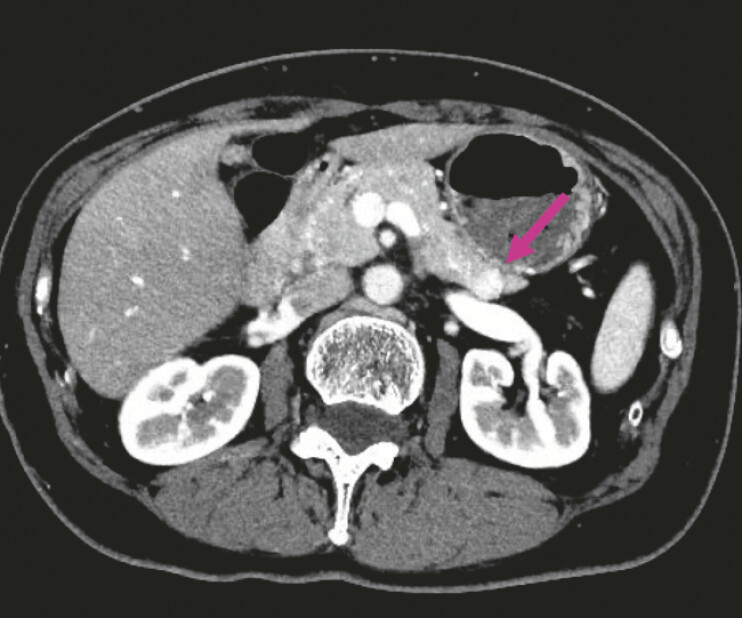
Contrast-enhanced computed tomography demonstrated a 10-mm tumor (arrow) in the pancreatic tail with early enhancement.
